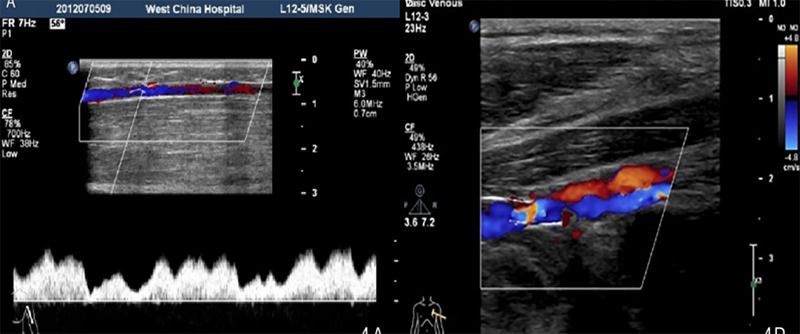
(A) Doppler assessment of pulse wave revealed thinning of the left axillary vein, (B)Left cephalic vein is thicker, draining into axillary vein.
